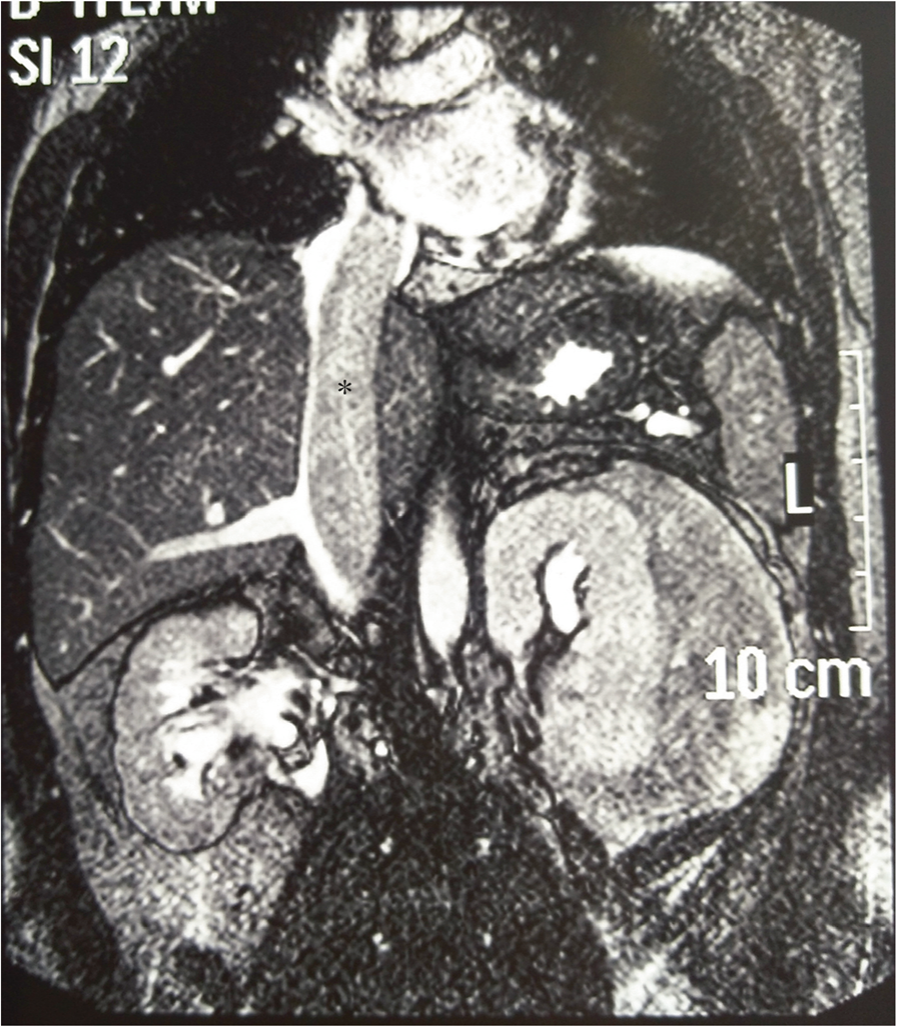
MRI of a left-sided RCC with right atrial tumor thrombus (*)
